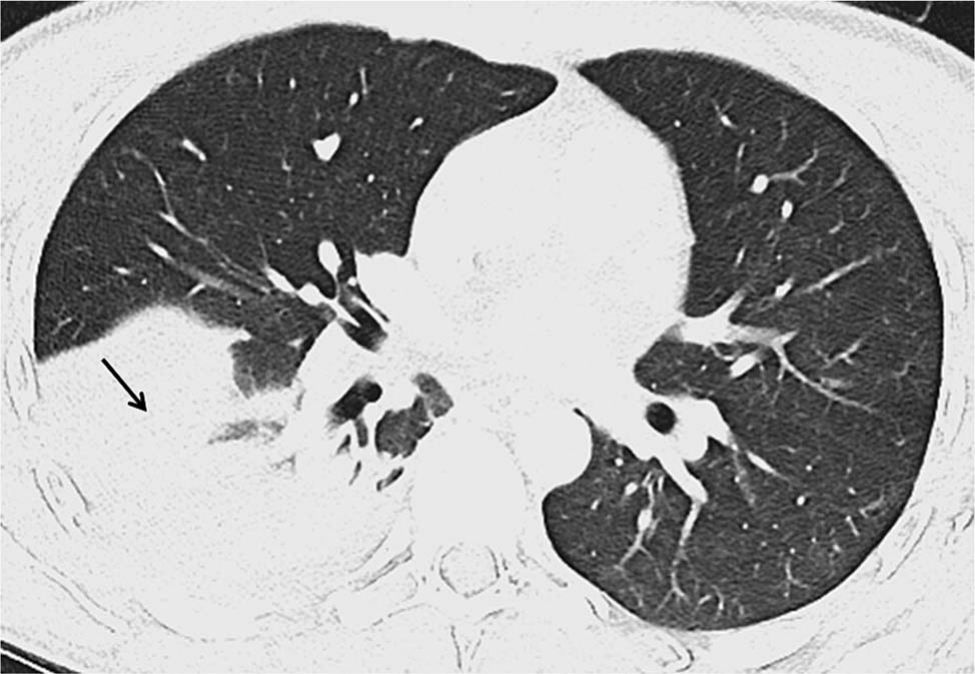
Thoracic computed tomography in axial view revealing dense infiltrates in the right lower lobe (black arrow)
